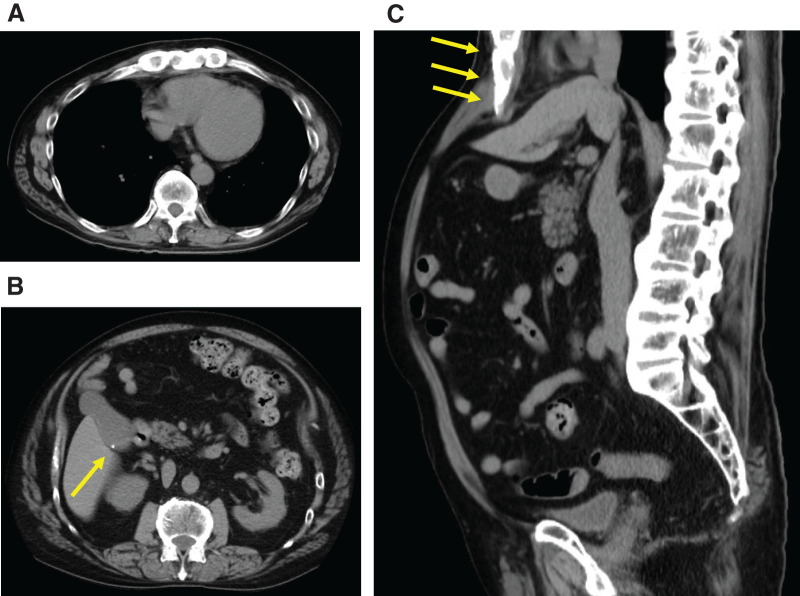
Abdominal multidetector CT images, axial views (A, B) and sagittal view (C). (A) Bony fusion changes were observed in the ribs and thoracic vertebrae. (B) Small calcified structures were noted within the gallbladder (yellow arrow). No gallbladder wall thickening or increased density of surrounding fat tissue was observed. (C) Bony fusion changes were observed in the thoracolumbar vertebrae. The liver was compressed by the lower margin of the thoracic cage (yellow arrows).
